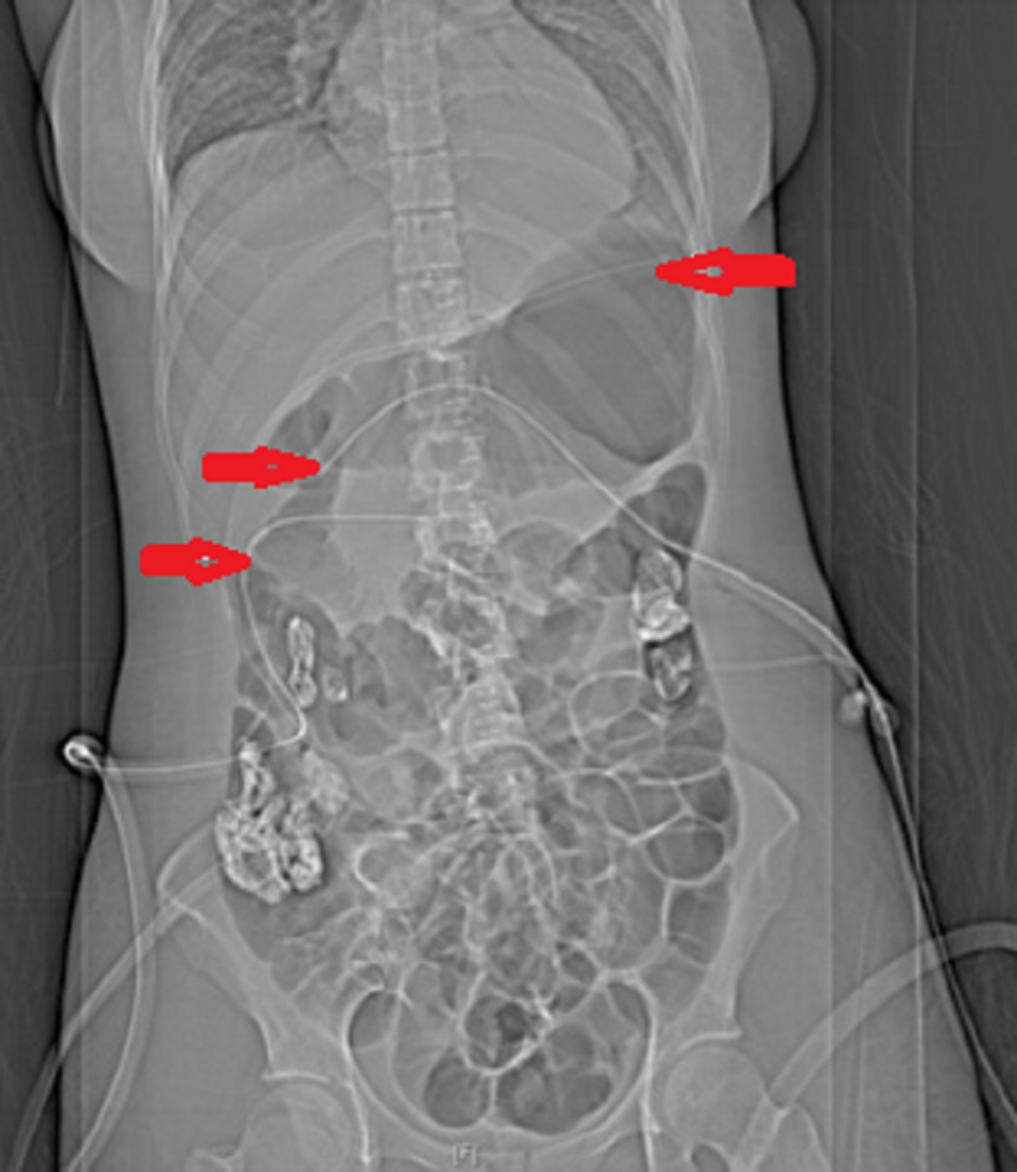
Post-operative abdominal plain X-ray demonstrating position of drains. Arrows show three large closed suction drain sites: Uppermost arrow - adjacent to the pancreas Second arrow - the subhepatic space Third arrow - the subphrenic spaces
